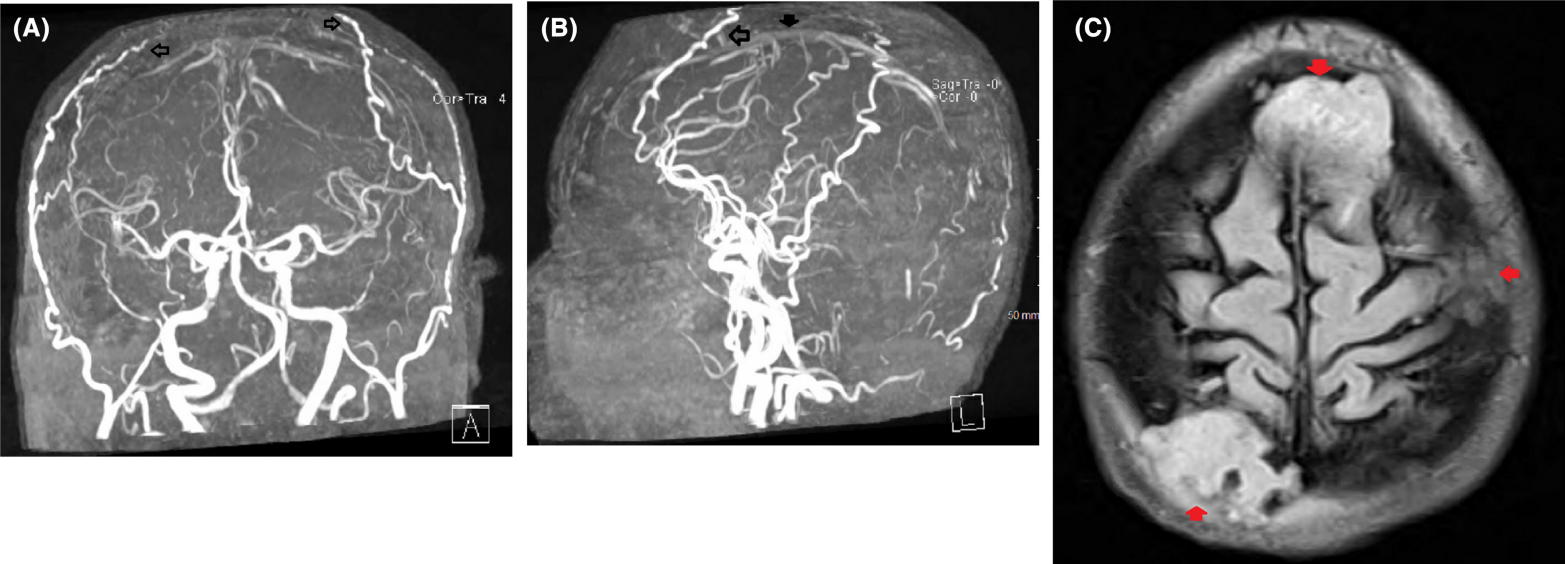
(A) Preoperative MRV (arterial phase) coronal sequence demonstrating the feeders from bilateral superficial temporal artery. (B) Preoperative MRA (arterial phase) sagittal sequence demonstrating the feeders from bilateral superficial temporal artery. The sagittal sinus appears to be compressed (filled arrow). (C) Preoperative MRI brain with and without contrast axial T2 sequence revealing extra‐axial lesions (bregma, larger right parietal, and the smaller left parietal).
